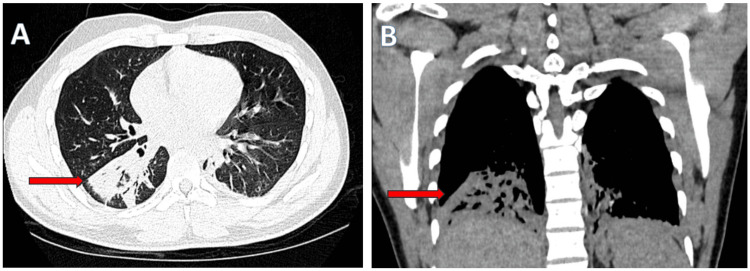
HRCT chest showing consolidation in the right lower lobe. (A) Axial and (B) coronal HRCT images of the chest showing a consolidation with air bronchograms in the posterior basal segment of the right lower lobe (red arrows). These findings are consistent with lobar pneumonia. HRCT: high-resolution computed tomography
